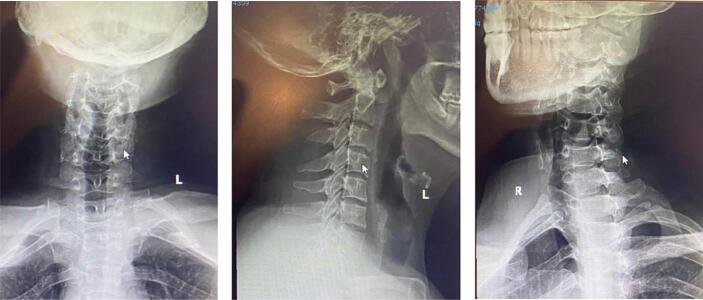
Cervical X ray. X-Ray on admission showed loss of cervical lordosis.
